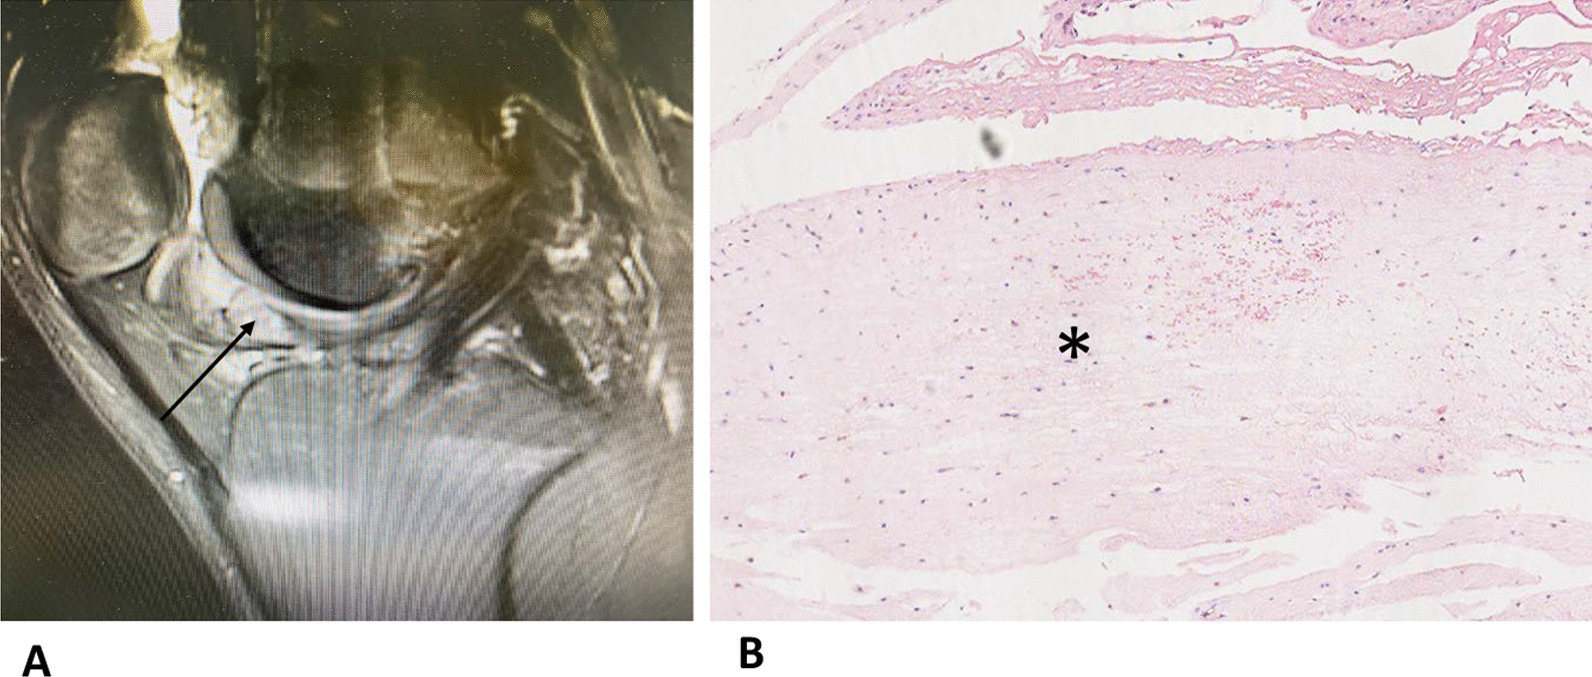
A MRI showing a cyclops lesion after ACL reconstruction (arrow); B dense connective tissue resembling tendinous tissue was detectable in all cyclops lesions (asterisk)
